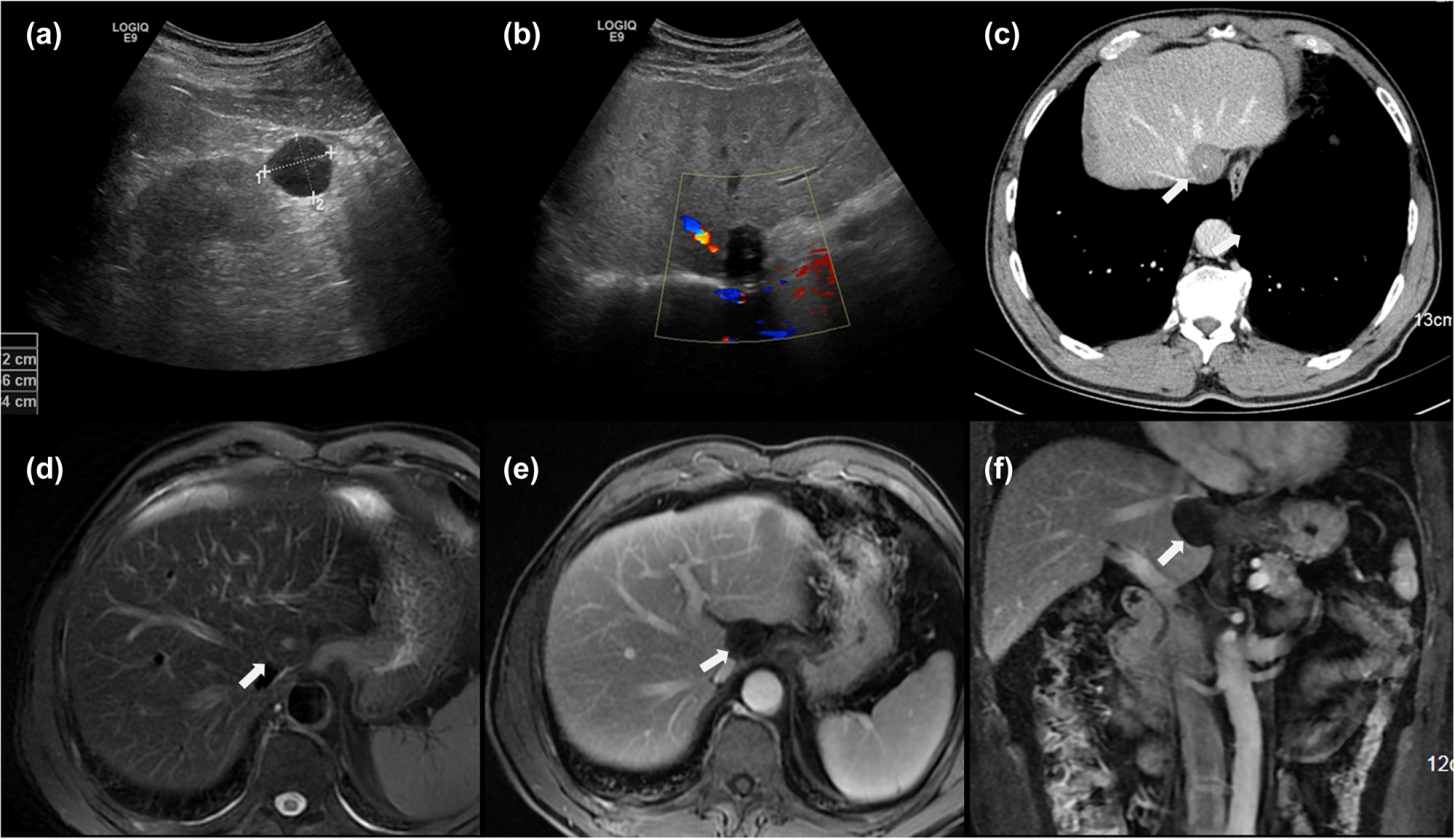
Doppler echocardiogram images (a and b) show a hypoechoic mass at the edge of the hepatic caudate lobe and no obvious blood flow signal in the mass. Contrast-enhanced venous phase CT image of the abdomen (c) reveals a concentric round, well-defined nonenhanced mass (arrow) with central calcification. Fat-suppressed T2-weighted images and gadolinium-enhanced fat-suppressed T1-weighted MR images (d–f) show a hypointense mass as muscle tissue without any enhancement (arrow).
